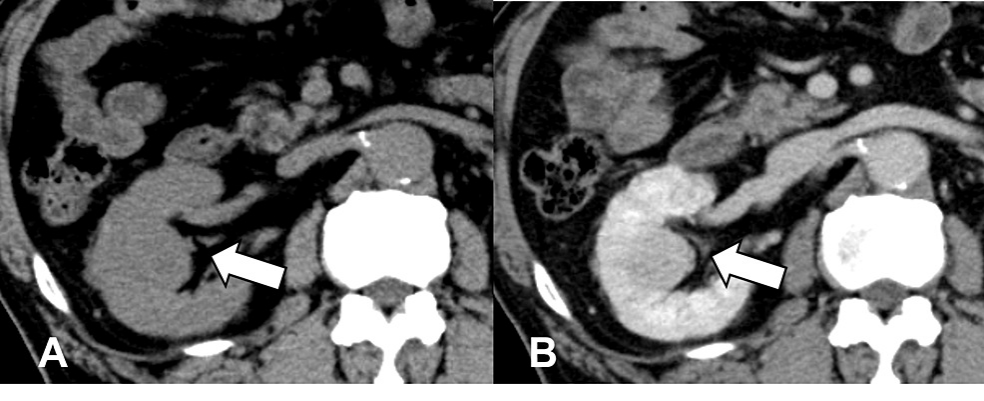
CT images of a 71-year-old man with clear cell RCC A: unenhanced image; B: enhanced image in nephrographic phase On axial UE-CT, a 2.8-cm isodense mass with unclear margins is seen in the right kidney (arrow). The mass protrudes inside the renal cortex like a column of Bertin. Pathologic examination confirmed that the mass was a 2.8 cm clear cell RCC protruding inside the renal cortex RCC: renal cell carcinoma; UE-CT: unenhanced computed tomography
